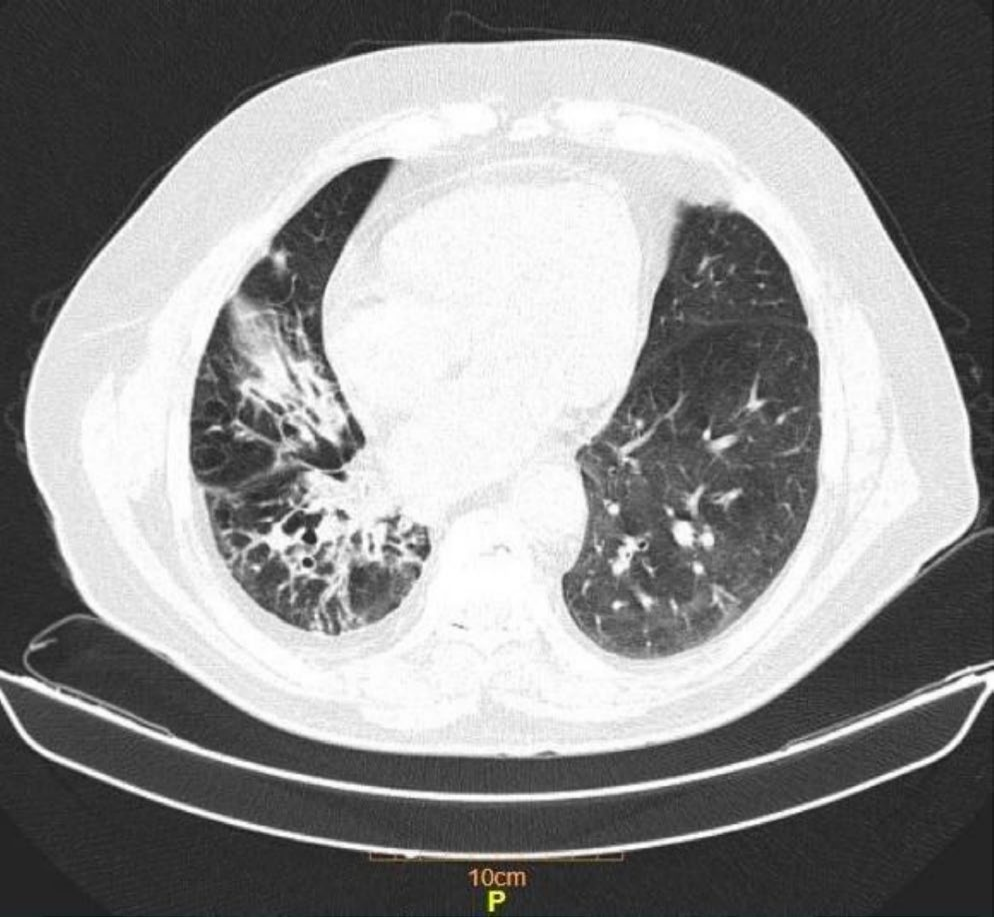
Section of spiral lung CT scan reveals mosaicism, right lower lobe bronchiectasis and trace right‐sided pleural effusion.
